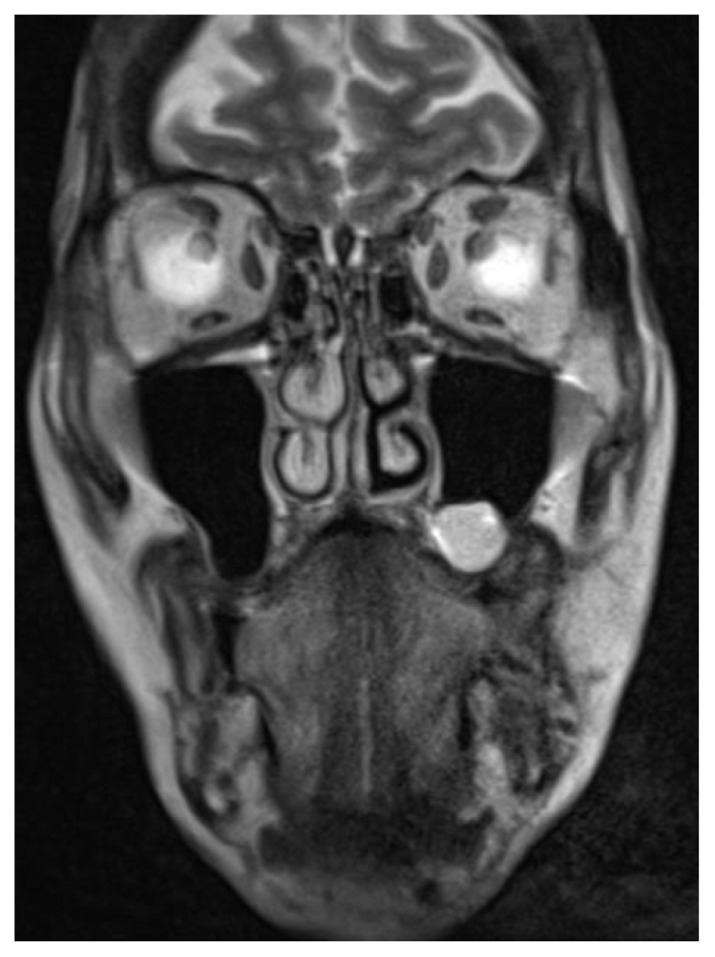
CT coronal image post-chemotherapy.
